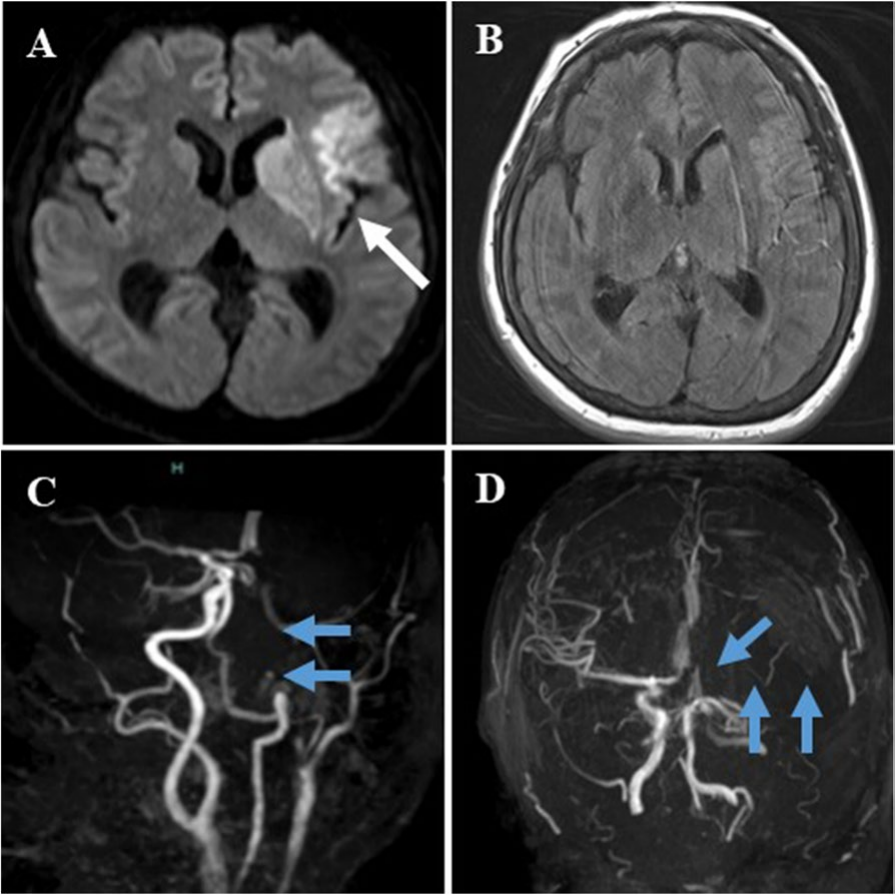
Postoperative MRI. A High-intensity area of patient’s left frontal lobe in the diffusion-weighted image (white arrow). B No intensity changes of her left frontal lobe in the fluid-attenuated inversion recovery image. C and D MR angiography showing total occlusion of her left internal carotid artery (blue arrows). MRI, magnetic resonance imaging
